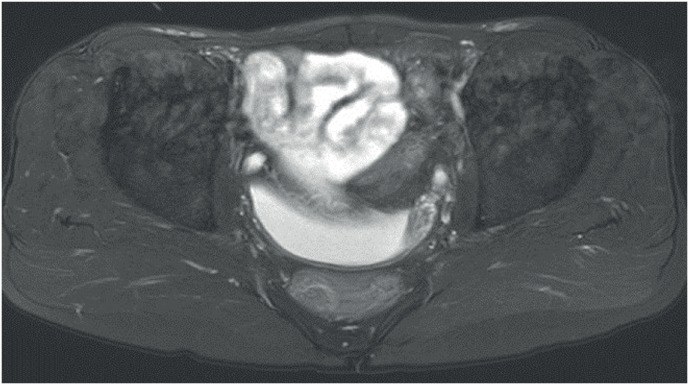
MRI showing the ureters anteriorly and the prostatic utricle cyst posteriorly. The bladder is absent.
